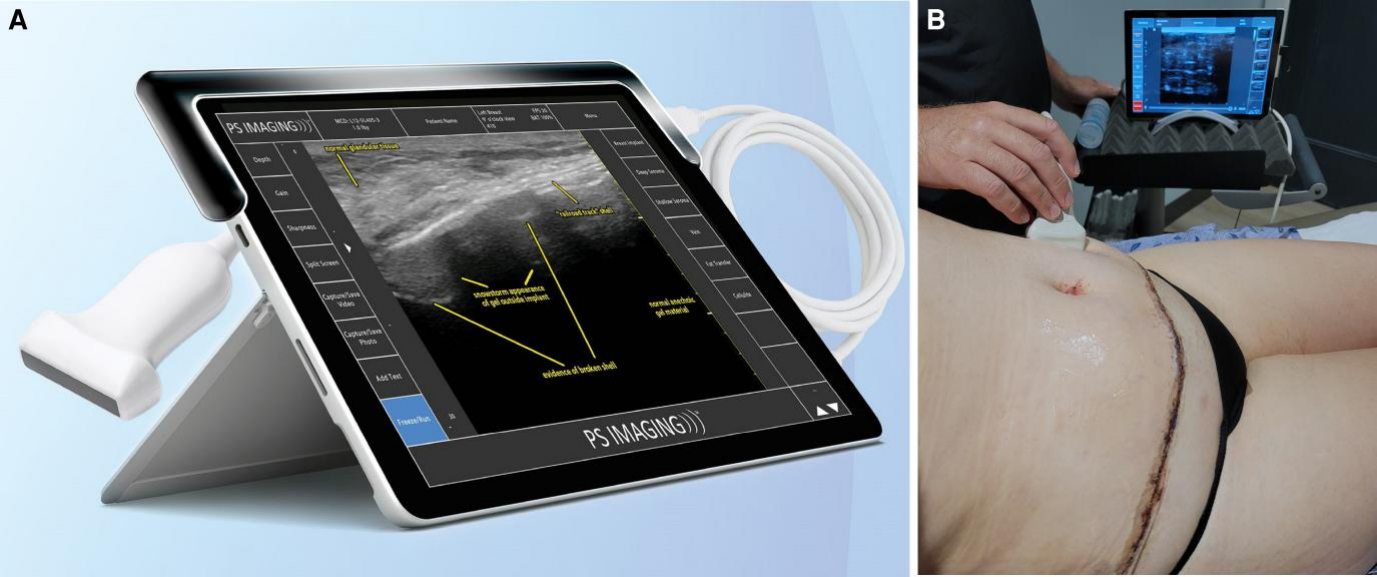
(A) Plastic surgery imaging high-resolution ultrasound imaging system utilized for the study with 12 MHz transducer. (B) Scanning a 36-year-old female patient's abdomen postoperatively on the day of Interi (Internal Closure System, IC Surgical, Grand Rapids, MI) removal.
